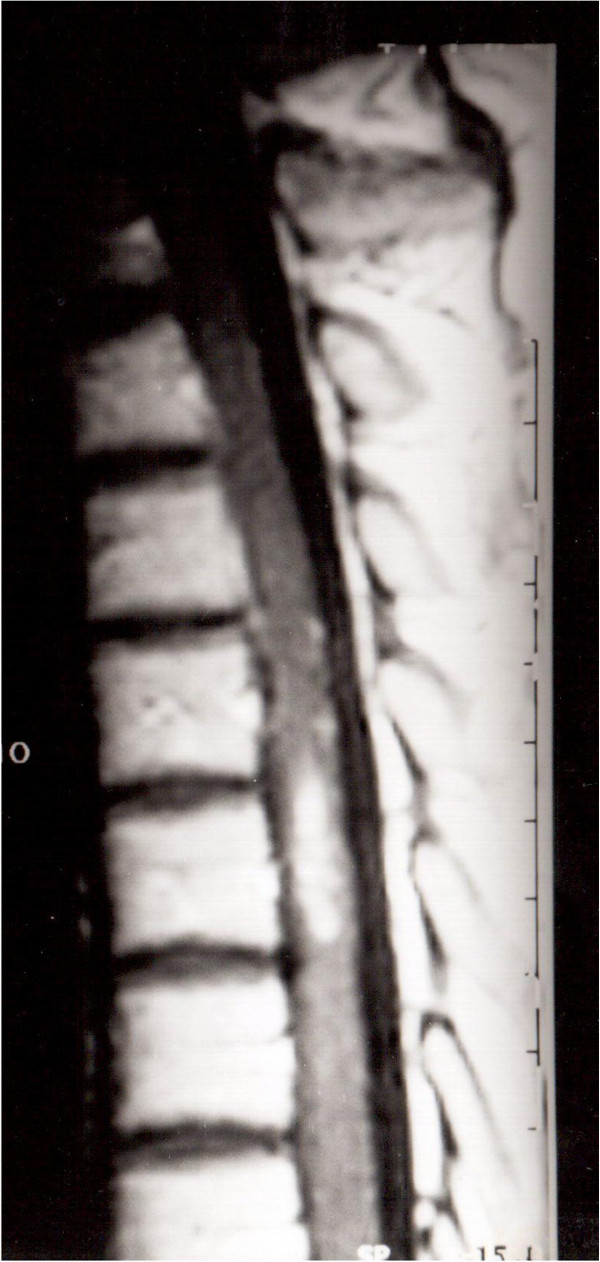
T1-weighted sagittal magnetic resonance image showing a high signal intensity mass at D5 level.
